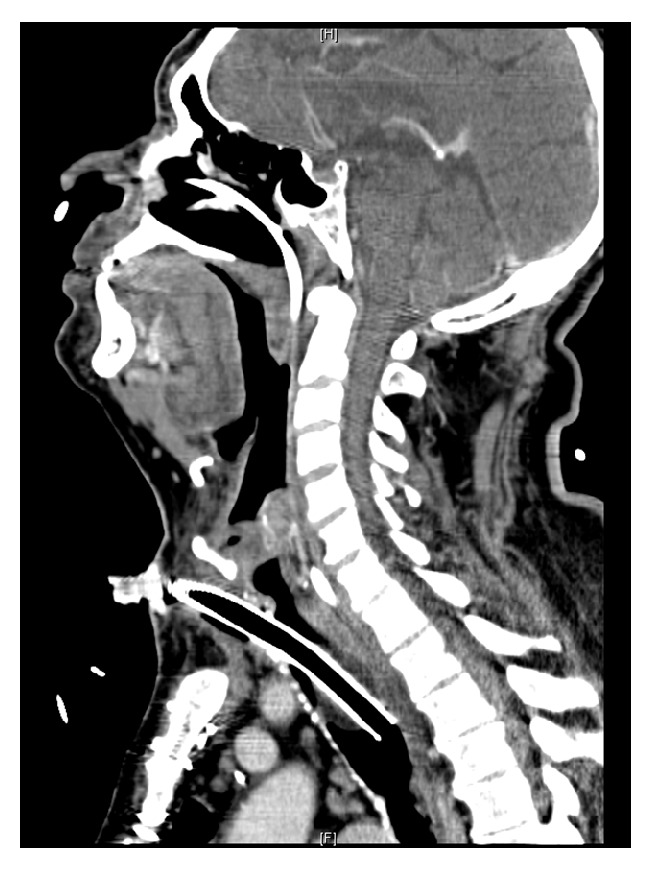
Postoperative Neck Soft Tissue CT revealing diffuse tracheal calcification.
